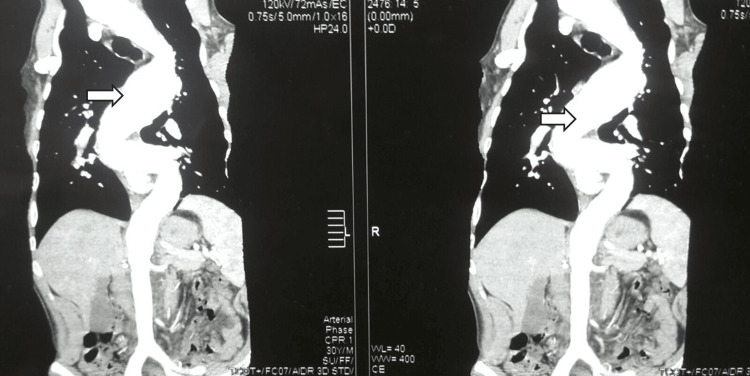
Arrows indicate elongation and tortuosity of thoracic aorta in CT aortography
